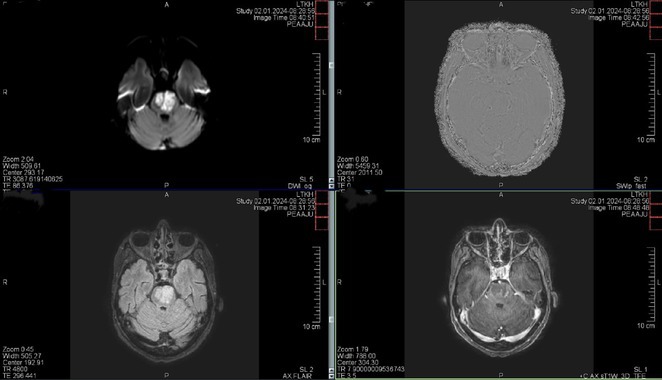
FIGURE 2 Third MRI scan showing ischemic lesion's progression in the brainstem.
